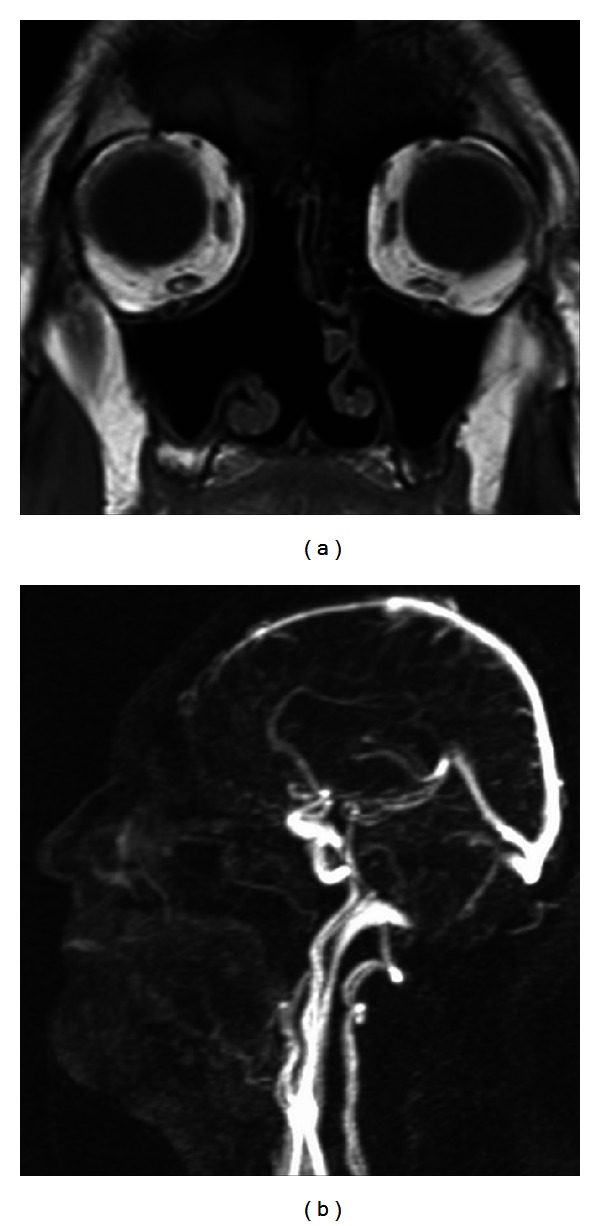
MRI (a) and MRI angiography (b) 12 months after surgery. The right nasal cavity appears to be free without any signs of tumor recurrence or residual.
